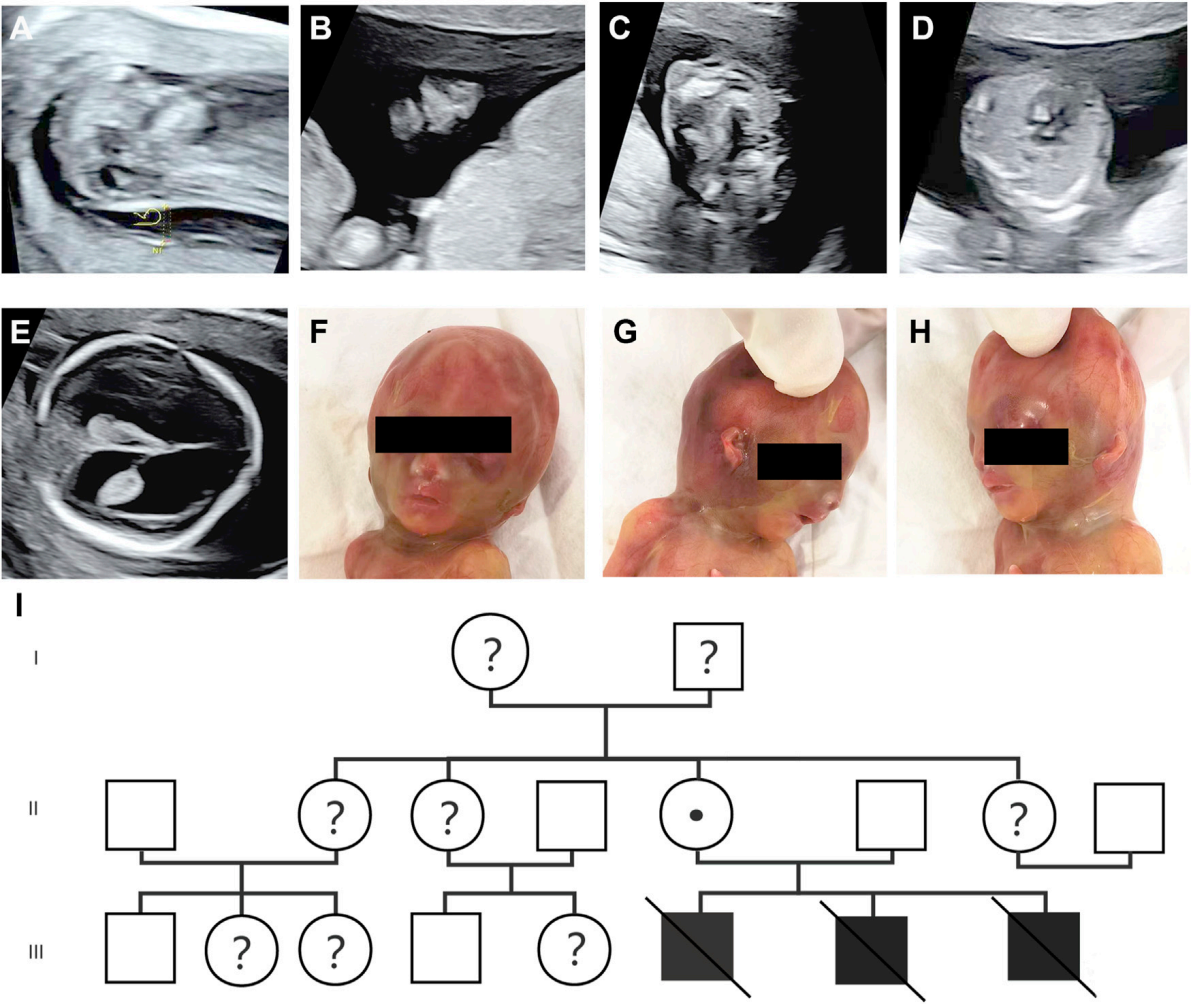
Prenatal ultrasound scan and fetal phenotype after delivery. (A–C) Prenatal ultrasound at 12+1 weeks of gestation showed thickened NT (6.5 mm) (A), nasal bone abnormalities (B), and cleft palate (C); (D,E) Prenatal ultrasound at 16+1 weeks of gestation showing VSD (D), and mildly enlarged bilateral lateral ventricles (E); (F–H) the image of fetus after delivery with a sunken nose and cup-shaped ears; (I) the lineage.
